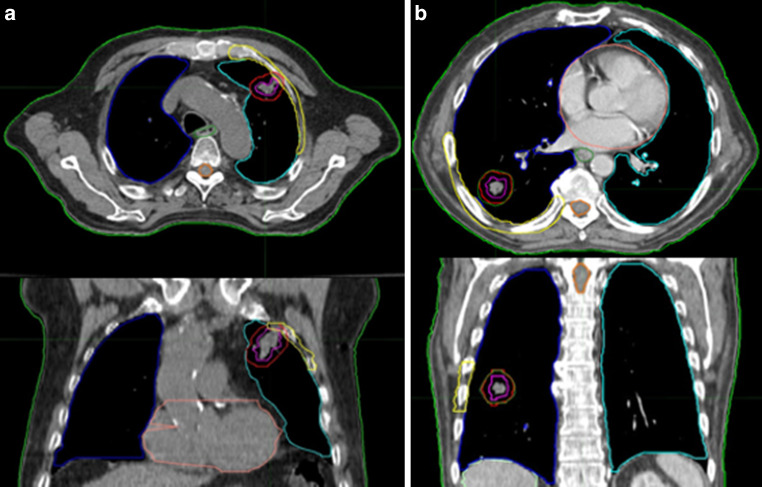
Axial and coronal CT slices with structures of the two patients used for this planning study
